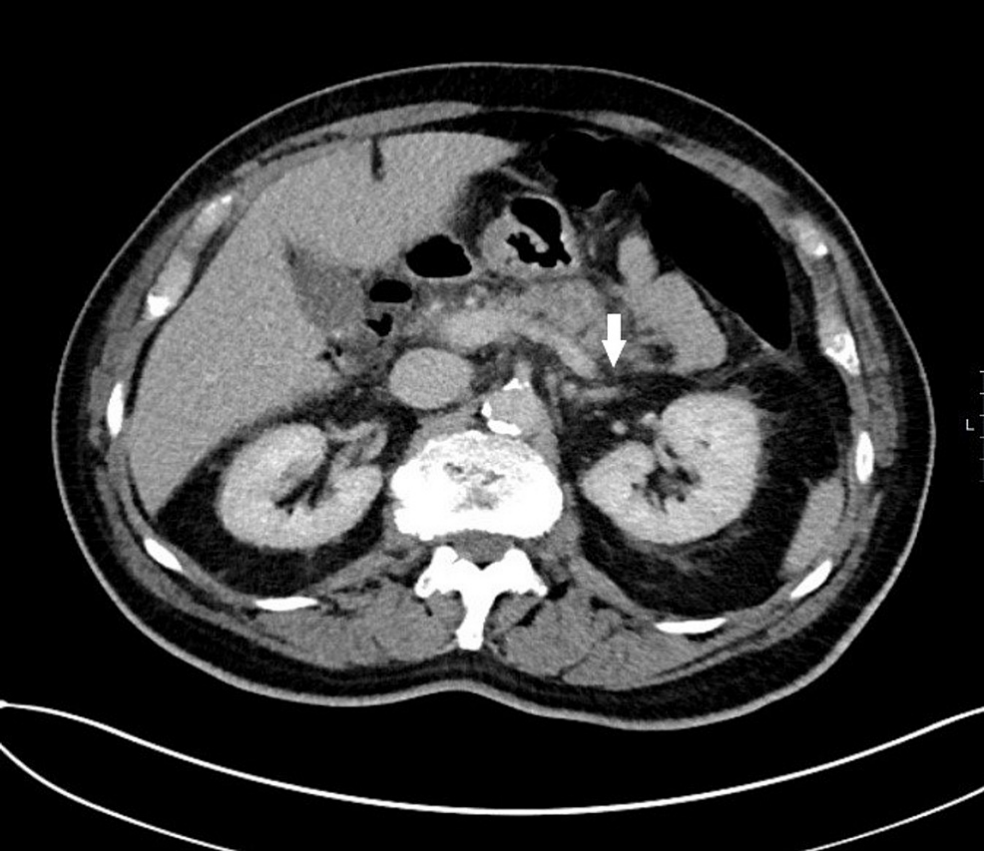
Axial CT scan showing acute pancreatitis. There is reduced enhancement in the region of the pancreatic tail and ill-defined pancreatic contours. Pancreatic parenchyma is not homogeneous in this region (white arrow).
